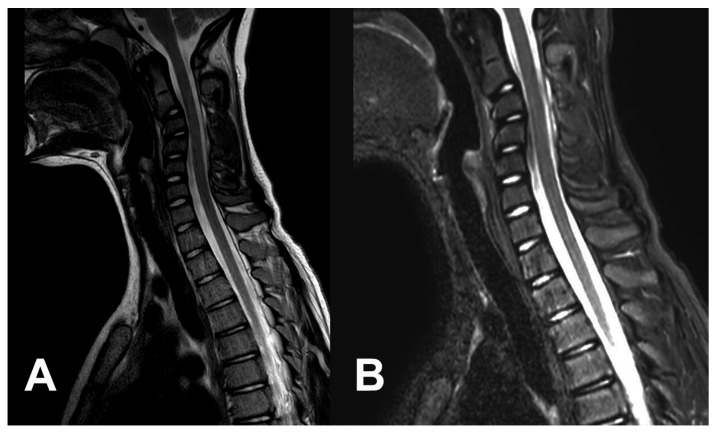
An MR scan performed three weeks after the injury shows that a kyphotic deformity is starting to form, but there are no direct signs of major disco-ligamentous injury. (A) T2-weighted sequences. (B) STIR-weighted sequences.
